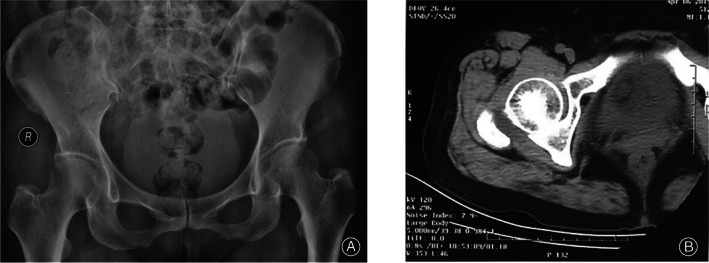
Imaging results. (A) Orthotopic X‐ray of the hip joint after surgery; (B) the postoperative CT of the hip joint, which suggested that the same lesion shown on X‐ray and CT before surgery was not found after surgery. The results indicate that the lesion has been removed by the surgical procedure.
